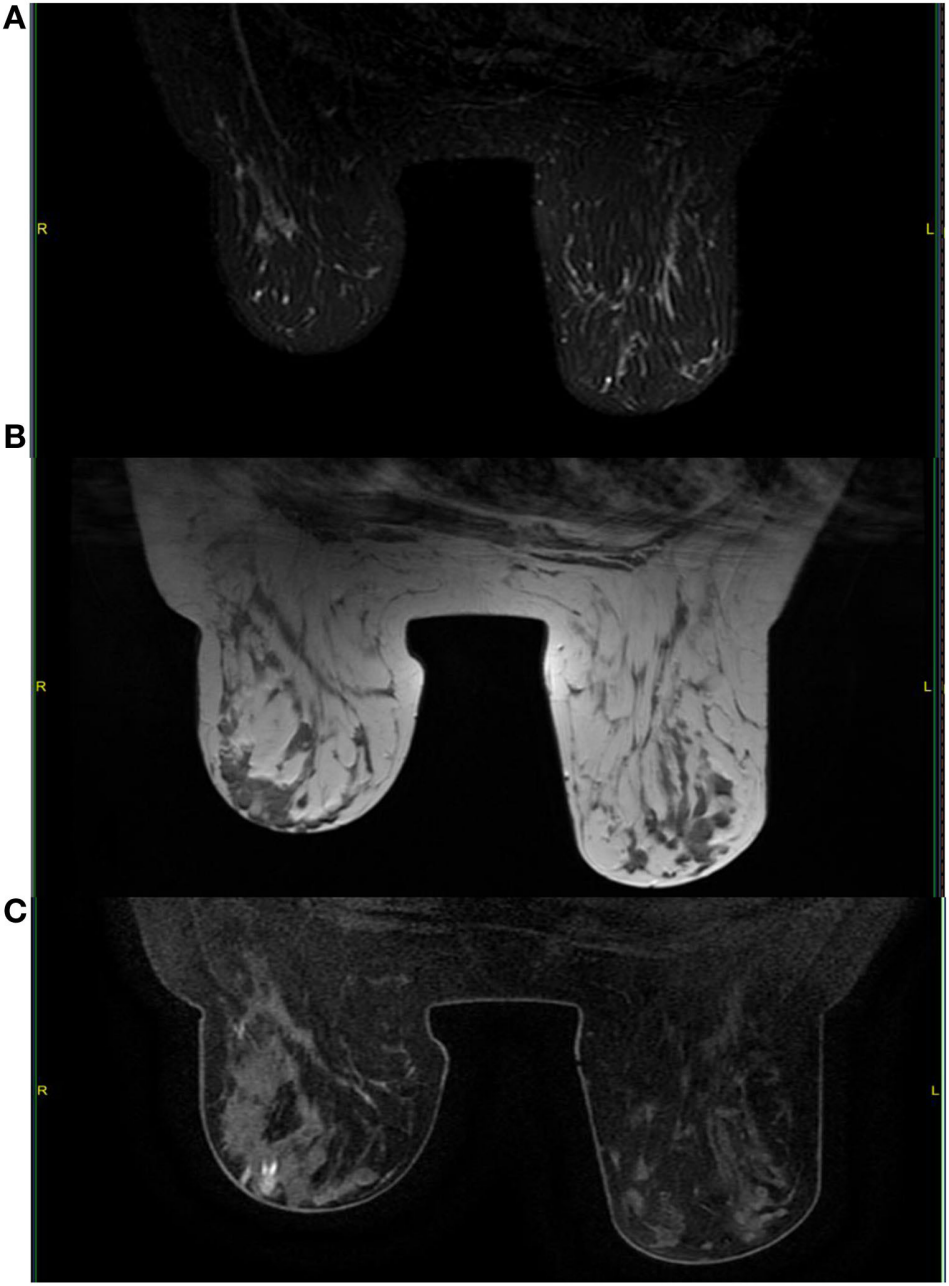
Follow-up with MRI shows significant decrease in tumor burden with no evidence of new lesions. A complete response was achieved in most lesions and observed by the absence of contrast enhancement especially in the big cluster of oval masses of the right breast (arrow). T2-weighted images with fat saturation (A); T1-weighted images (B); gadolinium-enhanced T1-weighted images with fat saturation (C).
